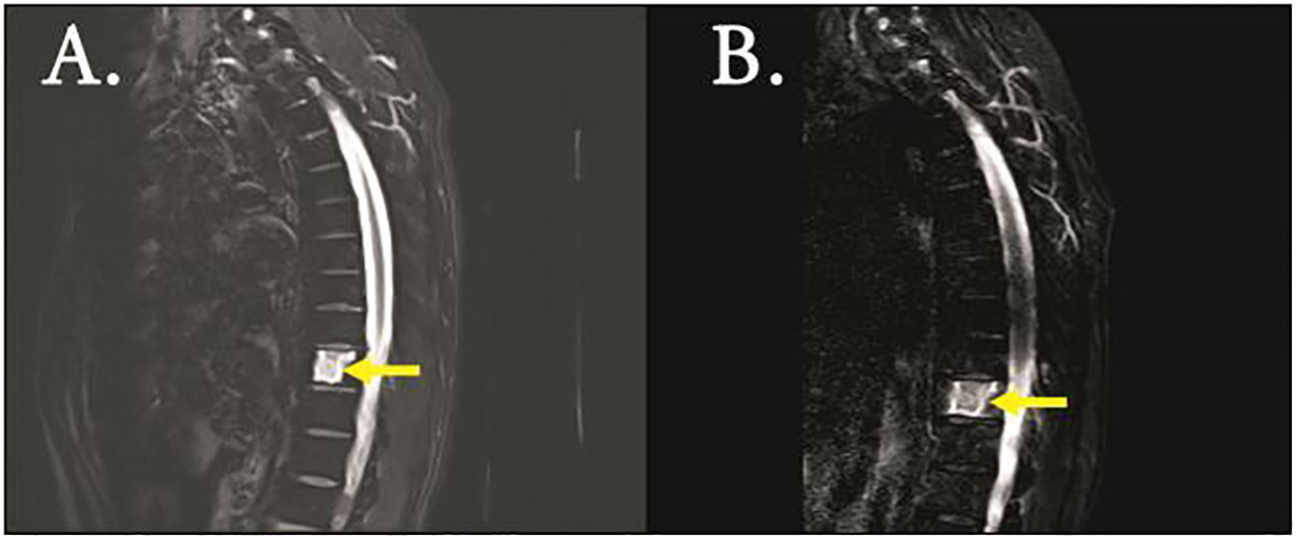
MRI (T2 imaging) revealed destruction of the thoracic vertebrae of initial diagnosis (A) and CR (B).
